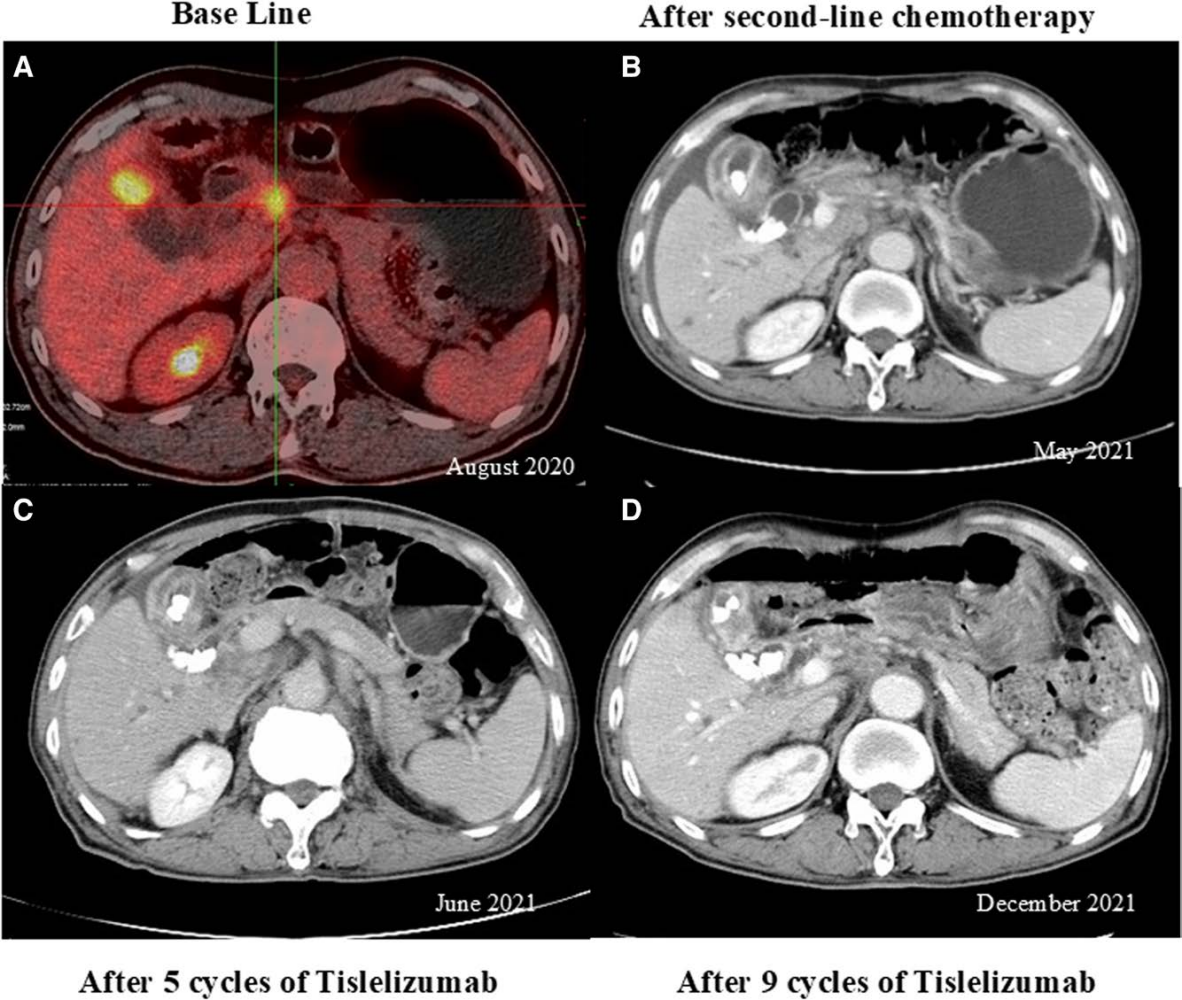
Radiological images of the patient. (A) Pretreatment PET/CT showing enlarged lymph nodes and a thickened gallbladder wall. (B) CT acquired after second-line chemotherapy showing a gallbladder mass and disease progression to the abdominal lymph nodes. (C) CT acquired after 5 tislelizumab cycles showing a reduction in the size of several lymph nodes. (D) CT acquired after 9 tislelizumab cycles showing lymph node enlargement indicating disease progression. PET/CT = tomography/computed tomography.
